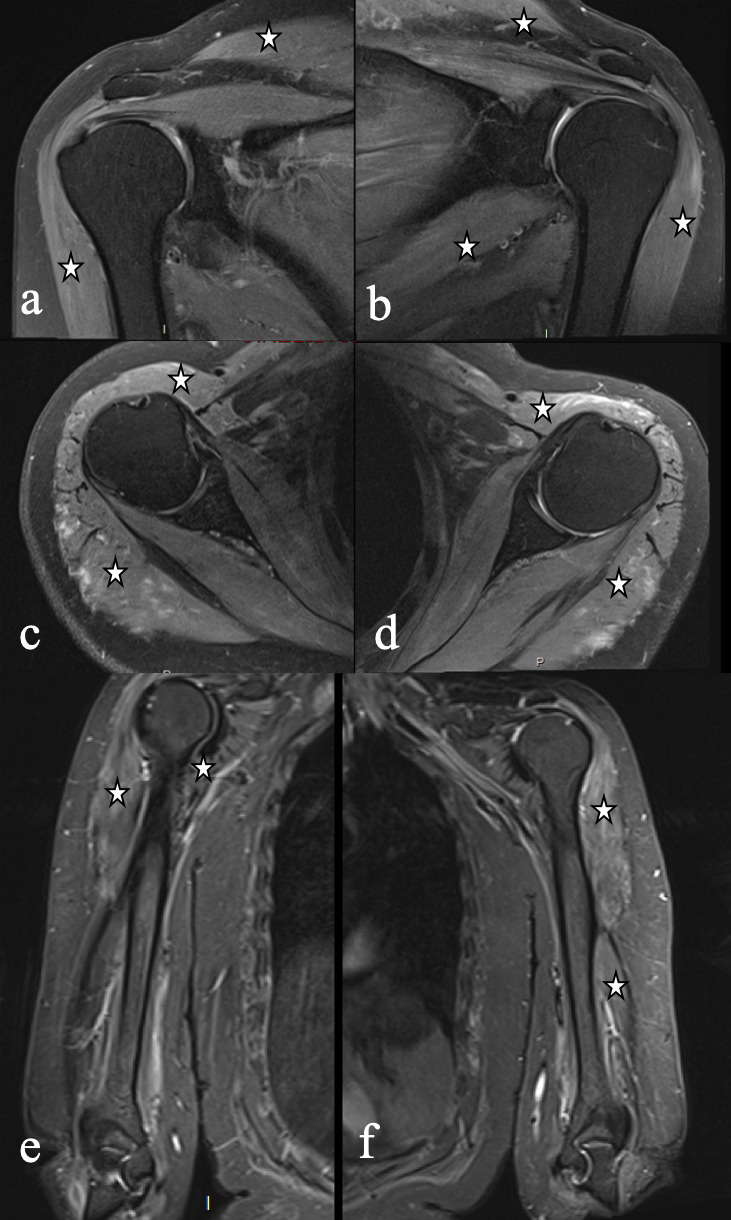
Follow-up MR imaging demonstrating reduction of muscle edema (white stars) after 4 months.
